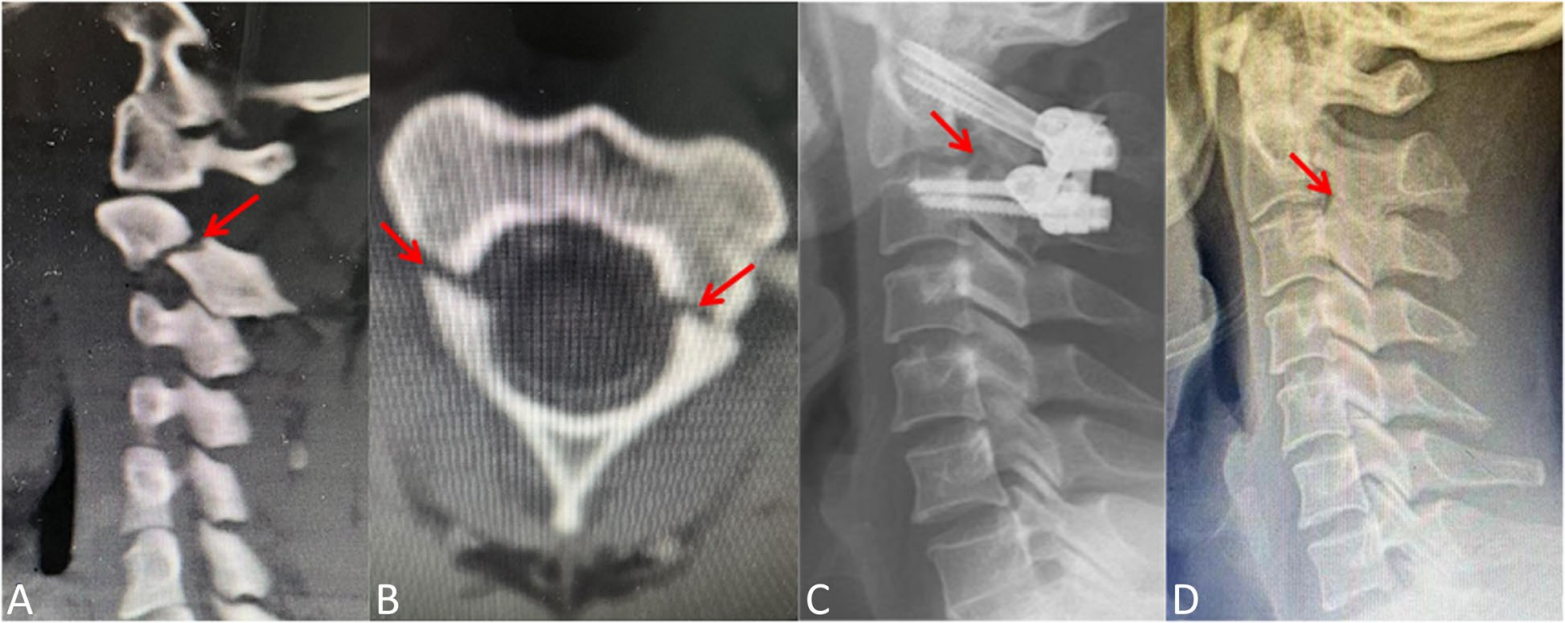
A 33-year-old man (case number 10) suffered from traumatic spondylolisthesis of the axis (Levine-Edwards type II), and he also underwent surgery of posterior fixation without fusion: A CT scan of parasagittal plane at the time of admission showing pars fracture of C2 on left side; B Axial CT scan showing bilateral pars fractures of C2; C Lateral x-ray on postoperative 7th day showing posterior C2-C3 pedicle screw fixation without bone graft was performed; D Lateral x-ray at 30 months following the initial surgery showing spontaneous fusion at bilateral C2-C3 facet joints (The patient underwent operation of removal of implants at 24 months after fixation surgery)
